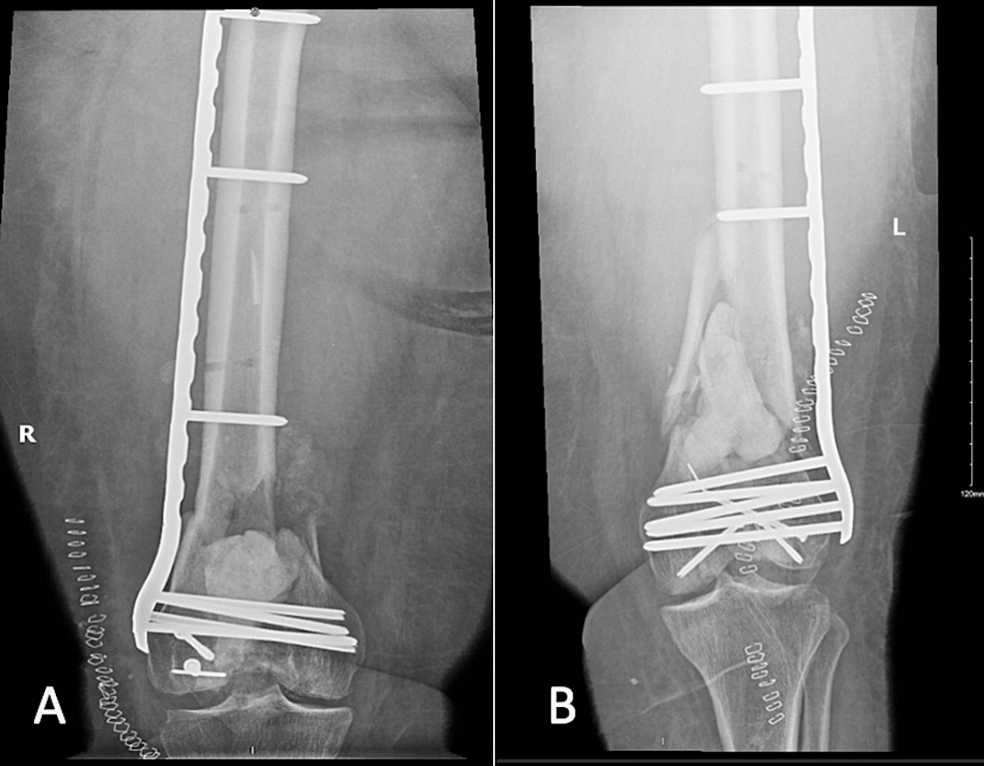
(A) Anteroposterior radiograph of the right distal femur. (B) Anteroposterior radiograph of the left distal femur.
